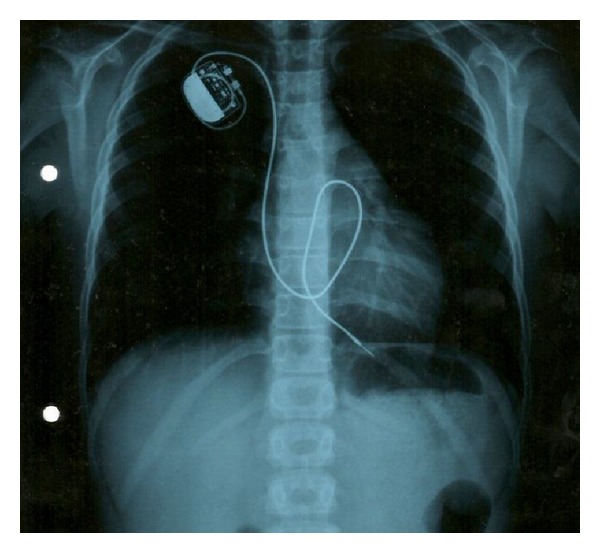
Thorax X-ray after VVI-R implantation.
